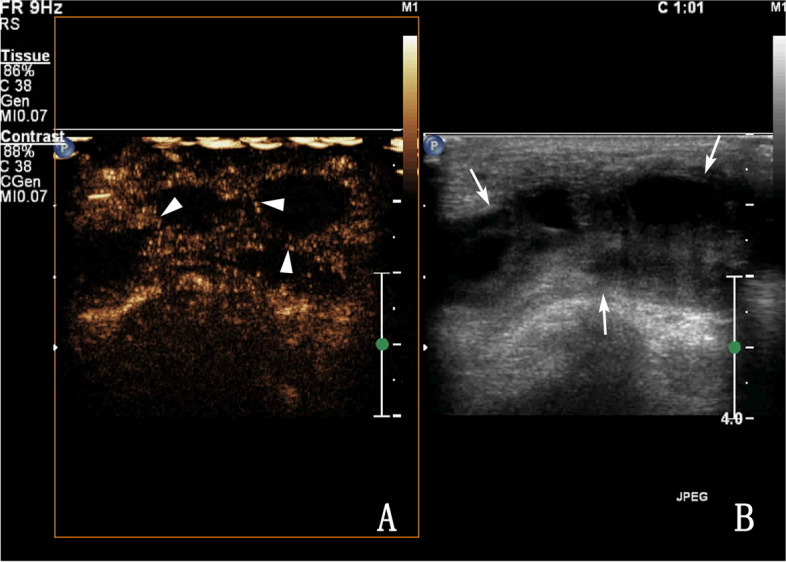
A 33-year-old male with tuberous vas deferens tuberculosis. A Routine ultrasonography showing a mixed echo-type lesion (coarse arrow), B Contrast-enhanced ultrasonography showing septal enhancement of the lesion (fine arrow), visible inside the irregular areas, not the enhanced area (type IIa)
